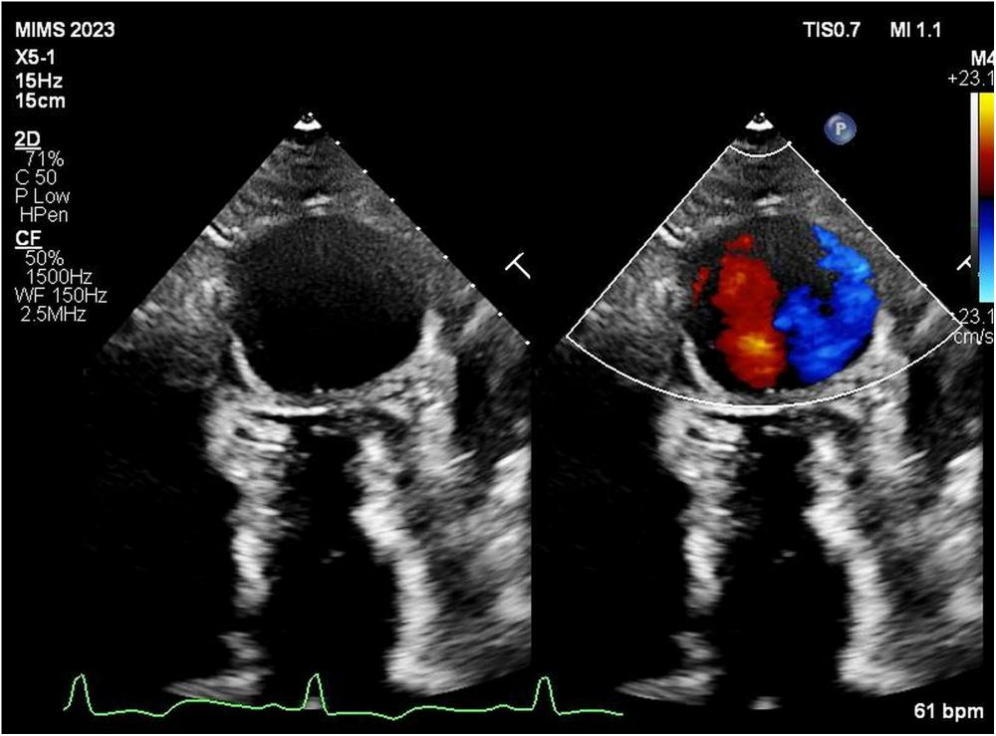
Colour flow Doppler imaging showing swirling flow within the aneurysm—the typical ‘Yin–Yang sign’.
